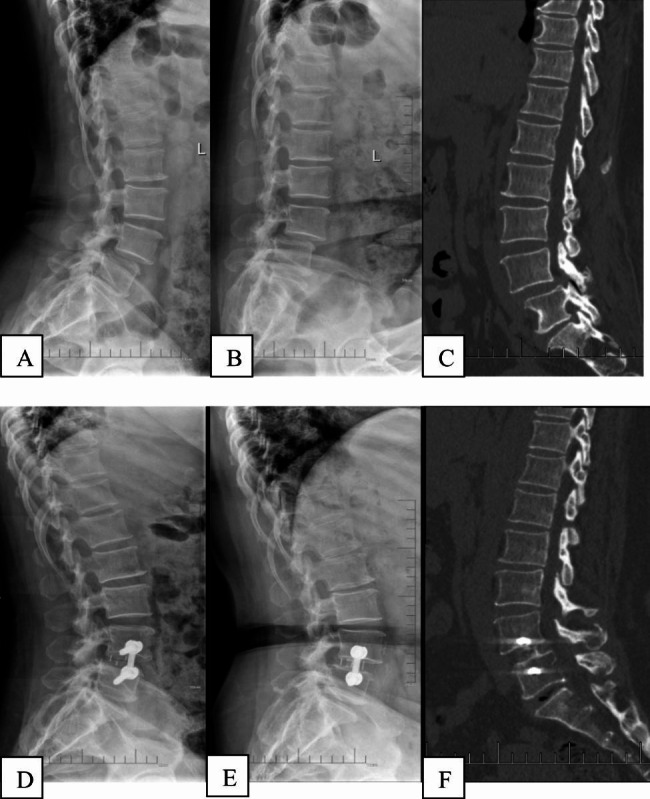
Images of a 56-year old female patient with normal bone density. (A)-(C). Preoperative lateral radiograph and CT show the L4/5 degenerative spondylolisthesis. (D)-(F). Postoperative lateral radiograph and CT showed the surgery provided reduction and fixation with satisfactory clinical results and relatively high fusion rate. (D:Postop 3d; E、F: follow-up at 1 year postoperatively)
